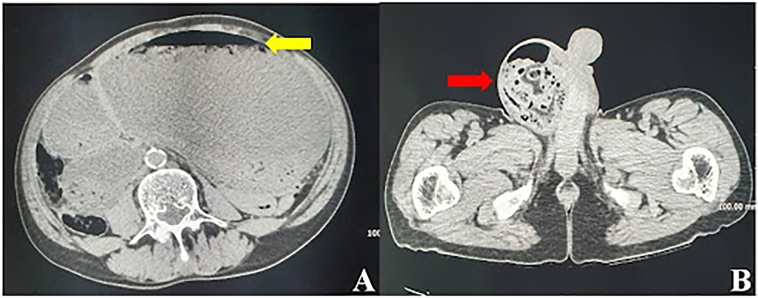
A,B. Preoperative abdominal computed tomography scan (transverse view) revealing massive gastroduodenal dilatation with pneumoperitoneum (Fig. 2 A, yellow arrow) and small bowel loops in the right inguinal hernia sac (Fig. 2 B, red arrow). (For interpretation of the references to colour in this figure legend, the reader is referred to the web version of this article.)
